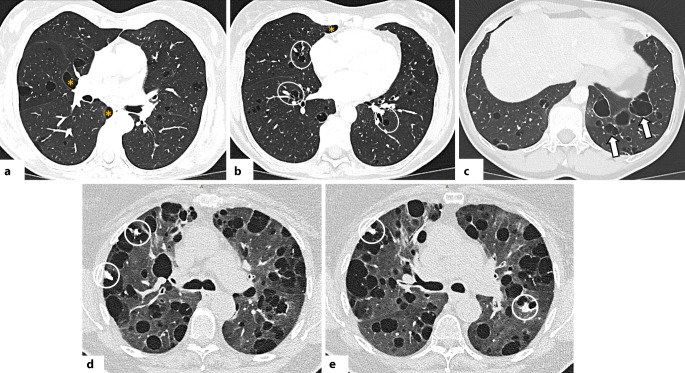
a–c Axiale Aufnahmen der hochauflösenden Computertomographie (HRCT): Muster einer lymphozytischen interstitiellen Pneumonie (LIP) bei einer Patientin mit Sjögren-Syndrom. Die Zysten sind dünnwandig und multiseptiert (*weiße Pfeile*), in zufälliger Verteilung und vorwiegend entlang peribronchovaskulärer Bündel (*weiße Kreise*) sowie subpleural bis hin zu mediastinalen Oberflächen lokalisiert (*gelbe Sternchen*). d, e Axiale HRCT-Aufnahmen bei einer Patientin mit Sjögren-Syndrom zeigen multiple verkalkte Noduli (*weiße Kreise*) in Kombination mit dünnwandigen Zysten, diese Befunde sprechen für eine pulmonale Amyloidose im Rahmen einer zugrundeliegenden LIP
